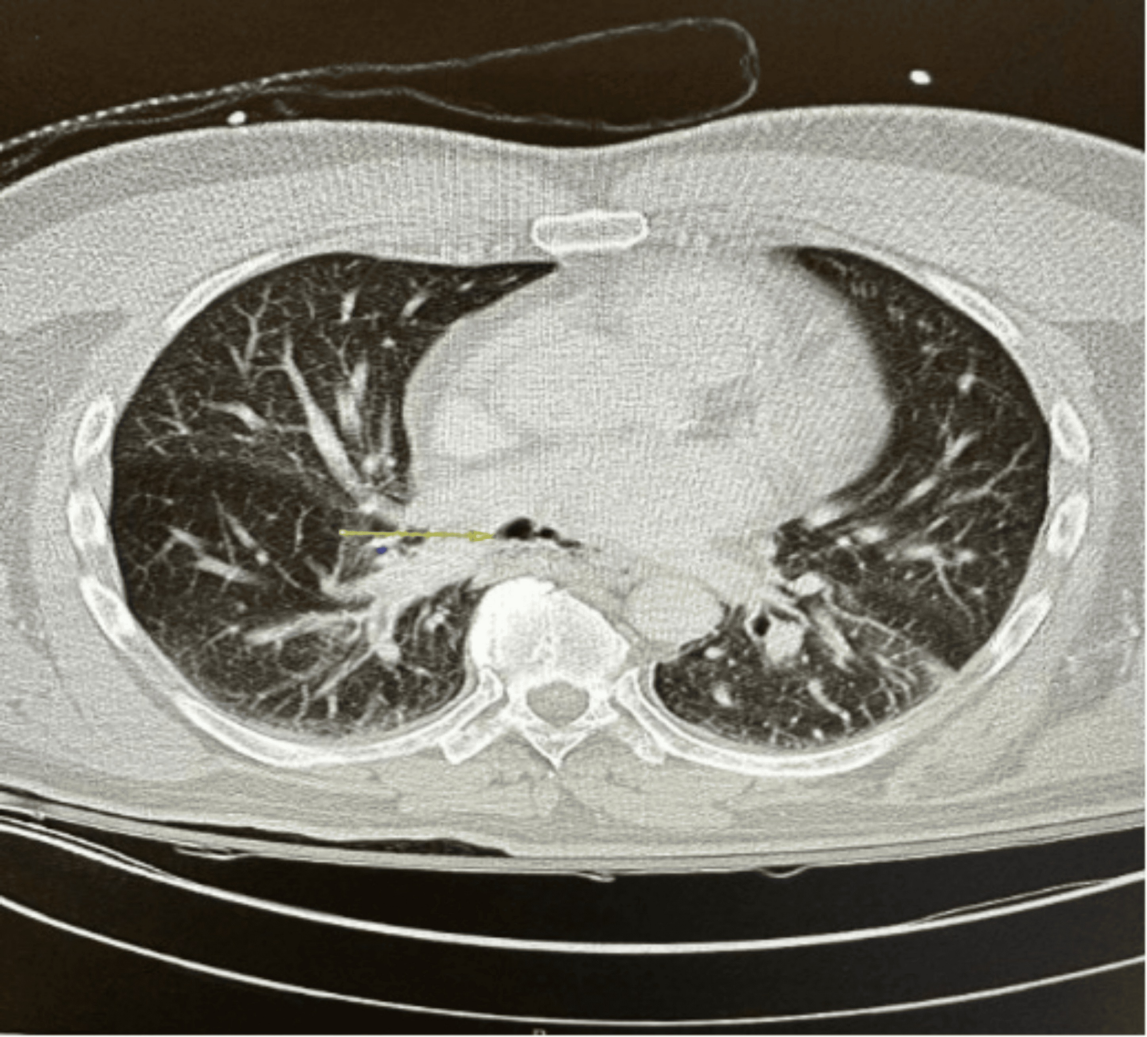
Axial thoracic CT scan: atrial-esophageal fistula marked by yellow arrow CT: computed tomography
